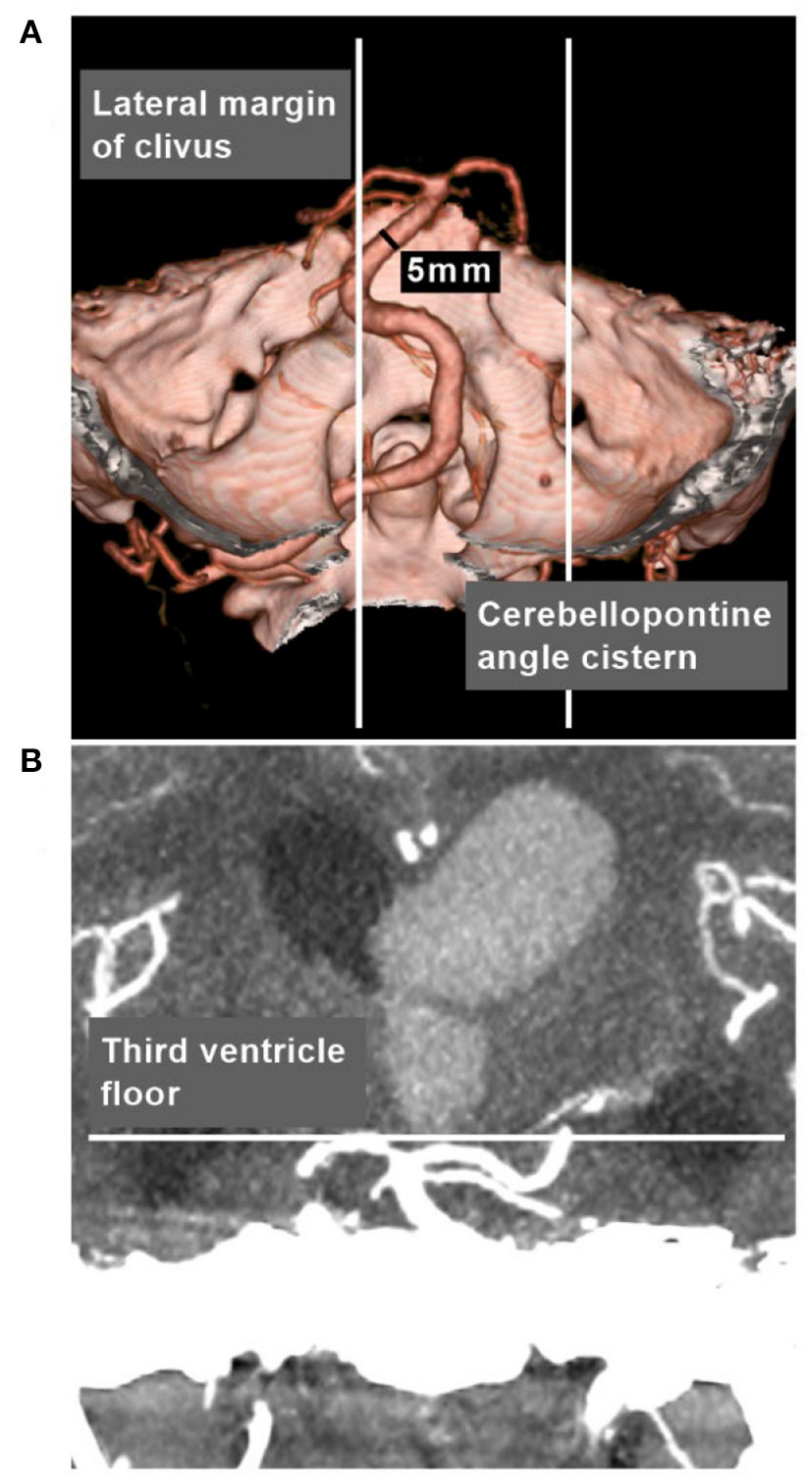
VBD definition in CTA. (A) CTA reconstruction image showing the vertebrobasilar arteries were >4.5 mm in caliber at the mid pons level of the BA, and laterally surpassed the lateral margin of the clivus. (B) MIP image showing that the BA tip reached the level of the floor of the third ventricle. BA, basilar artery; CTA, computed tomography angiography; MIP, maximum intensity projection; VBD, vertebrobasilar dolichoectasia.
